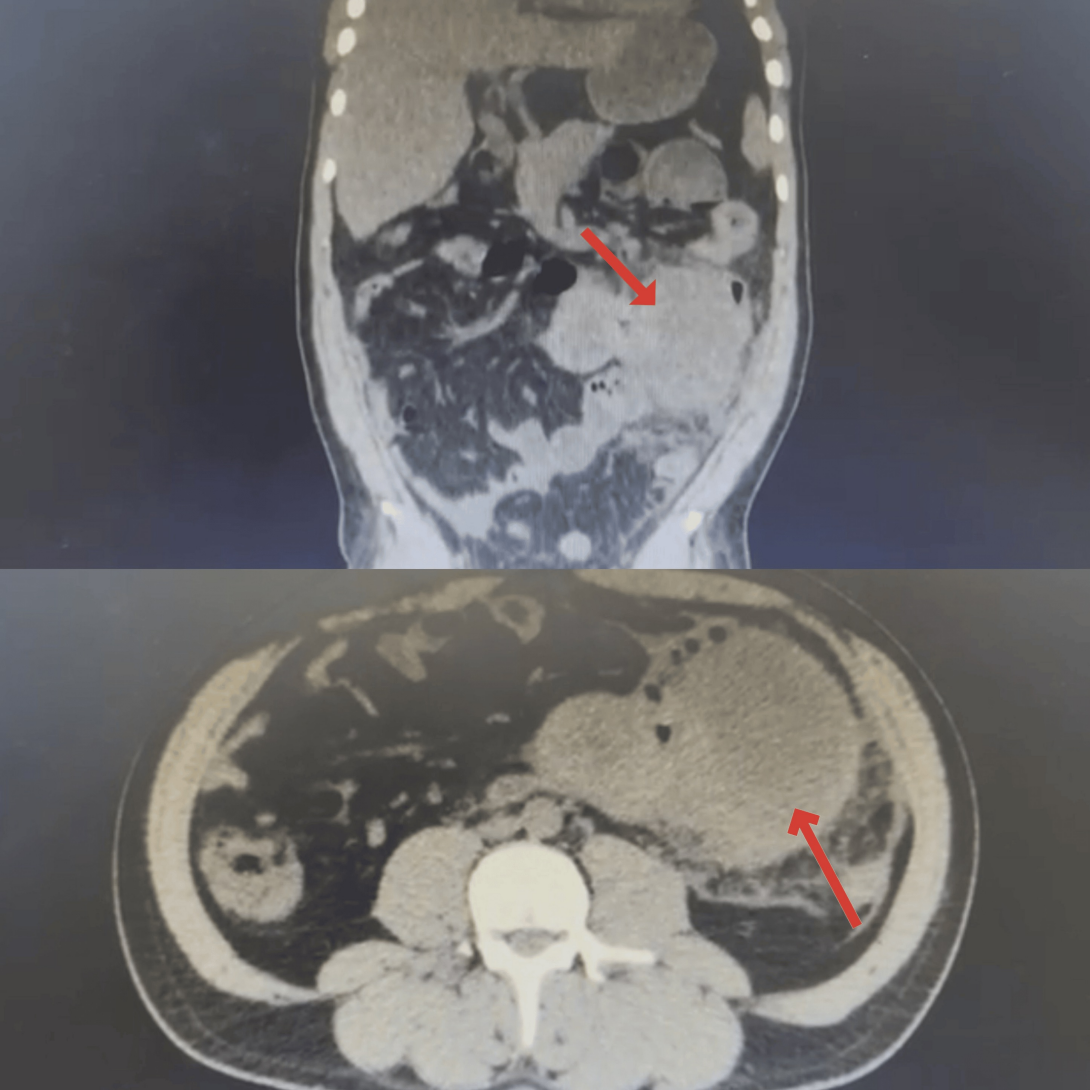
Coronal (upper image) and axial (bottom image) CT of the patient The CT of the patient showed an enlarged tumor of the small intestine (indicated by the arrows).
